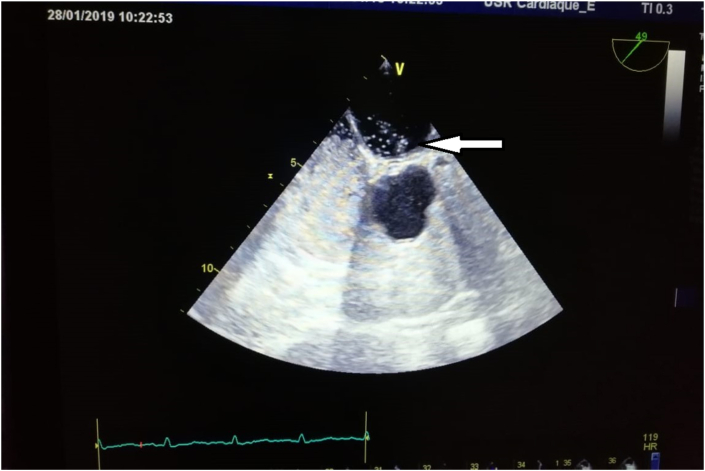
Transoesophageal echocardiography demonstrated positive bubble test.
